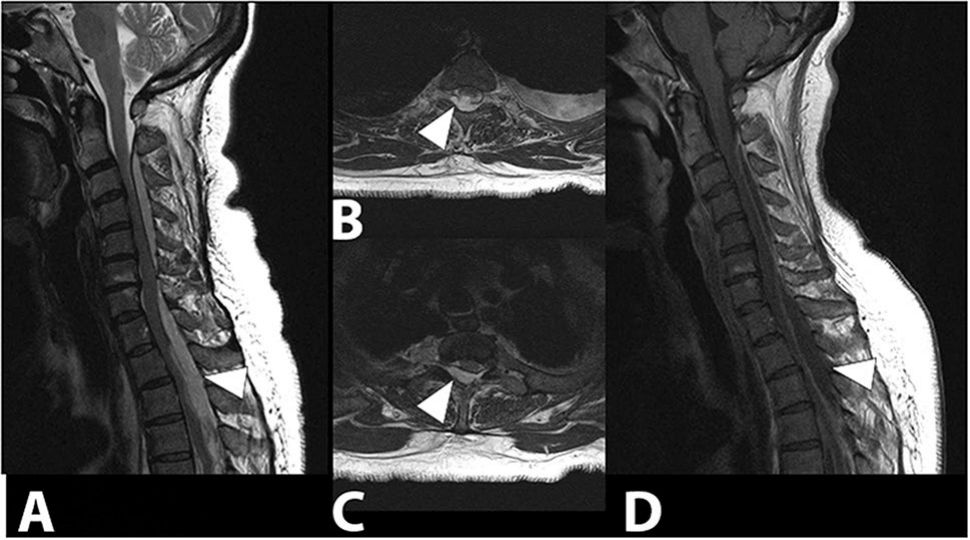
Sagittal (a) and axial T2-weighted images (b, c) and sagittal T1-weighted fat suppression image with contrast (d). Staphylococcus aureus posterior cervico-dorsal epidural abscesses that compress and displace the spinal cord anteriorly can be observed (arrowhead)
